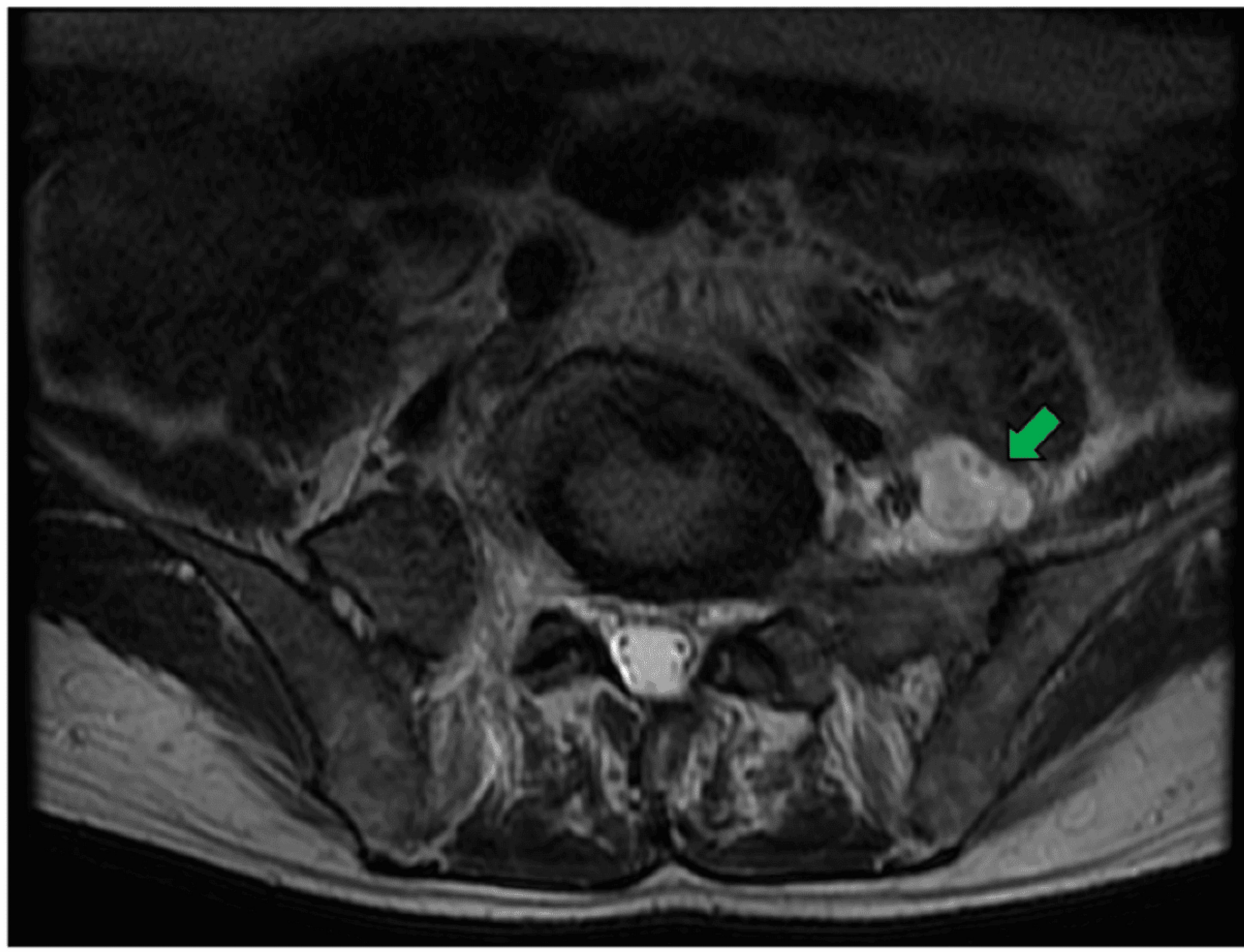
Contrast-enhanced MRI of the lumbar spine, axial view, T2 FRFSE sequence. The green arrow indicates a hyperintense collection consistent with an abscess in the left psoas muscle. MRI, magnetic resonance imaging; T2 FRFSE, T2 fast recovery fast spin echo.
